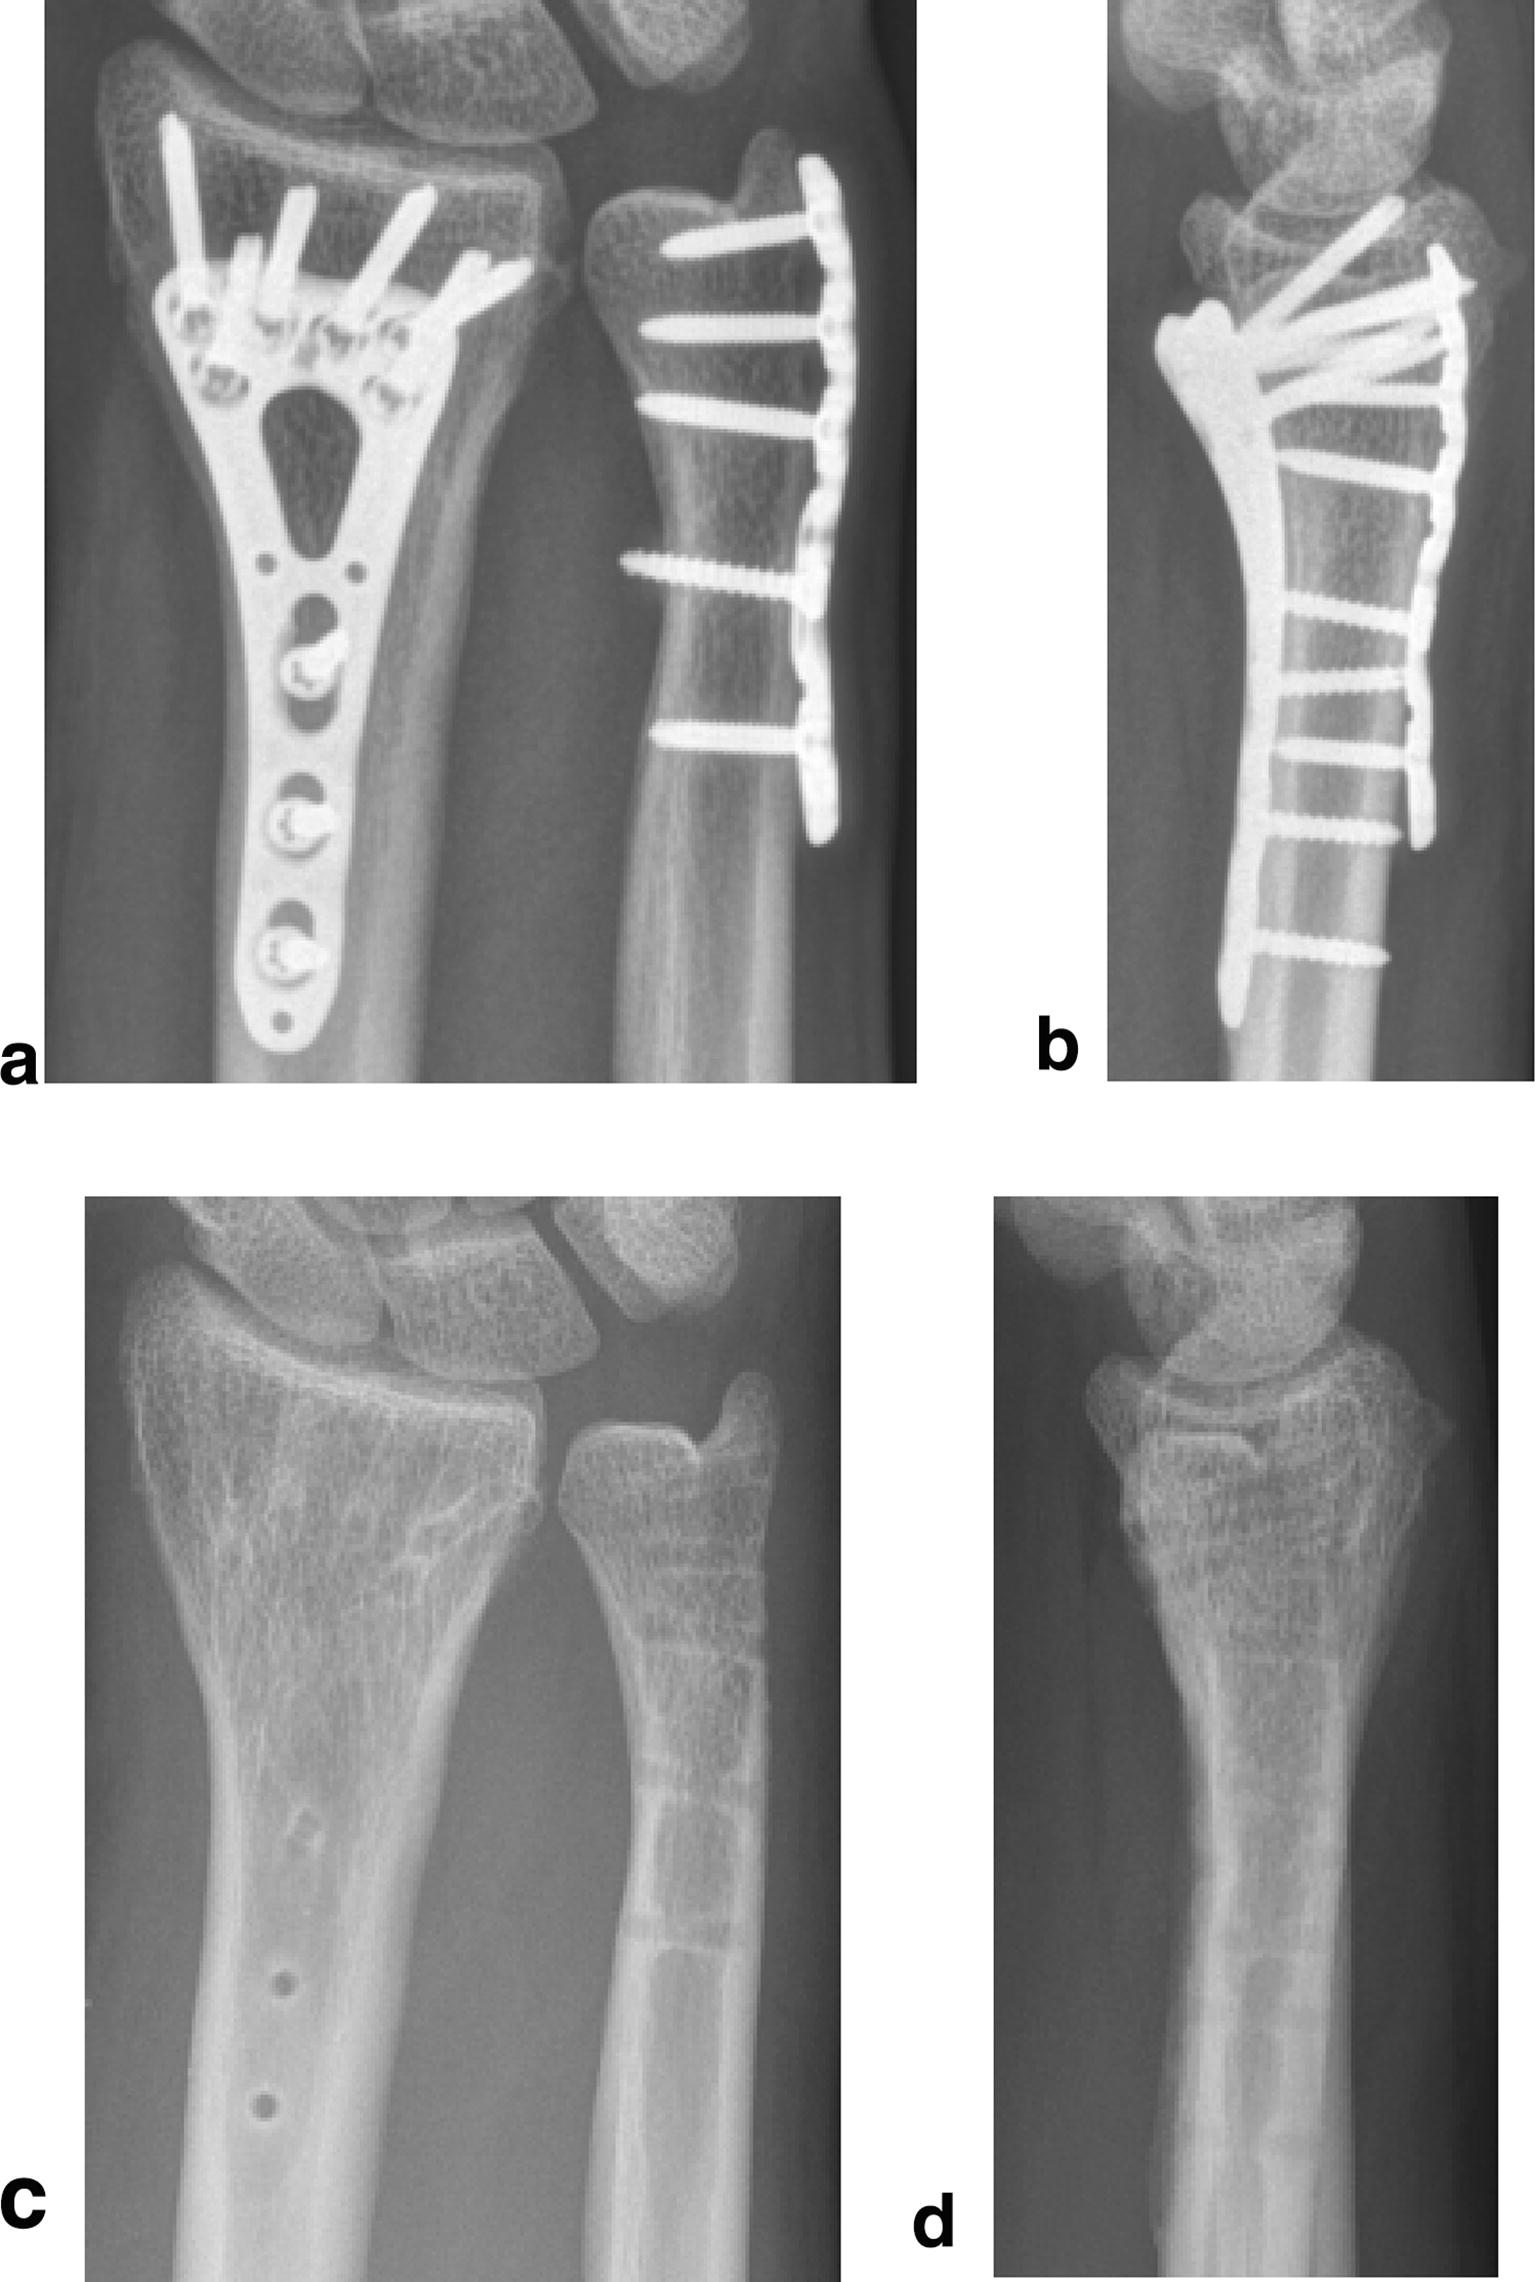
Follow-up imaging case 1. Radiograph (a antero-posterior view; b lateral view) demonstrating healed fractures 3 months postoperatively and radiograph (c antero-posterior view; d lateral view) demonstrating healed fractures after removal of the osteosynthesis after 1 year
